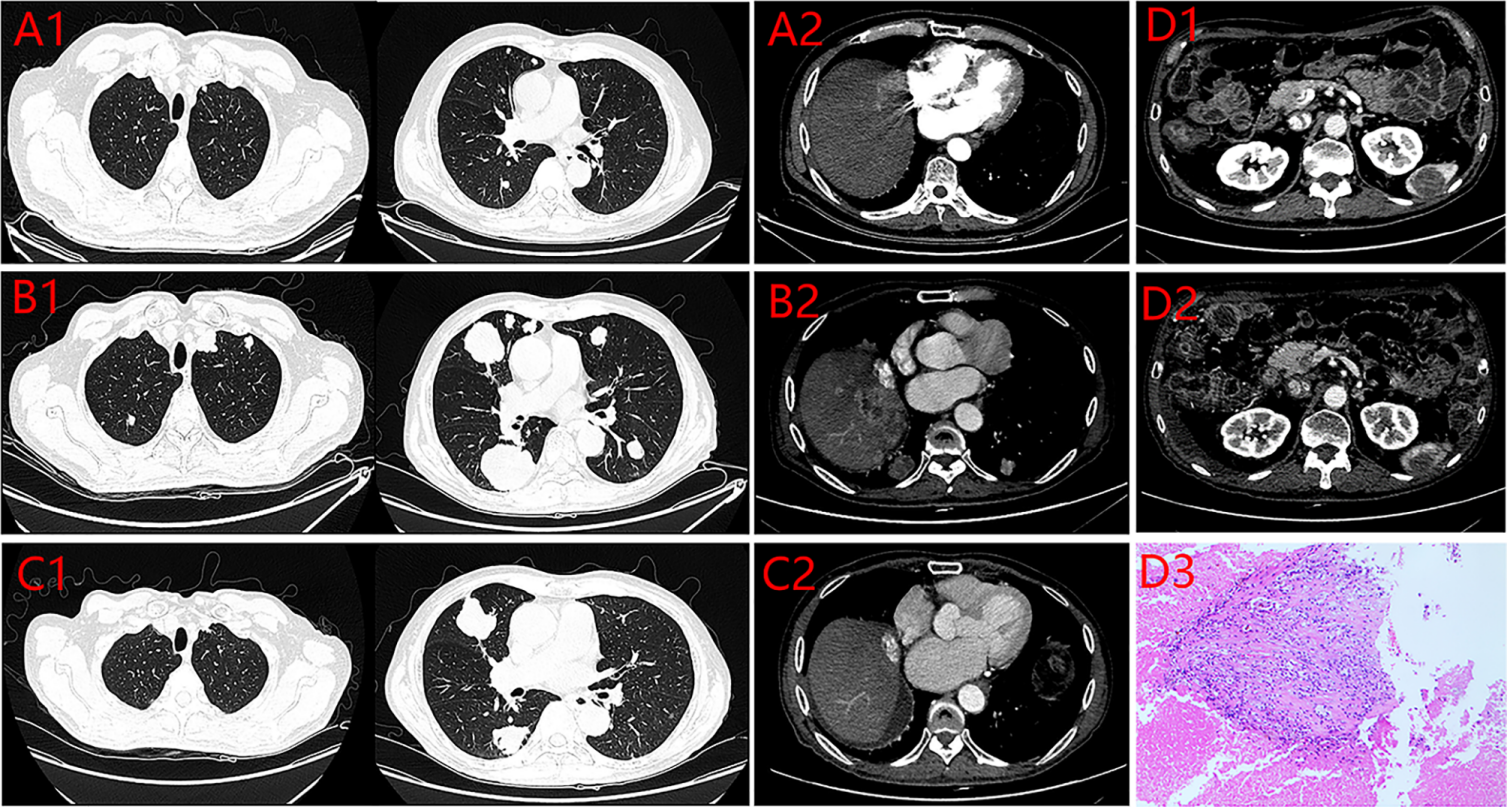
Enhanced CT of the lung and the abdomen. (A) 2017.09.17 (1. the Metastatic tumors of Lung 2. the Recurrent focus of Liver). (B) 2019.04.19 (1. The Metastatic tumors of Lung 2. the Recurrent focus of Liver). (C) 2019.11.11 (1. the Metastatic tumors of Lung 2. the Recurrent focus of Liver). (D) 1.spleen lesion (2019.4.19) 2. Spleen lesion after Pem plus Len (2019.11.11) 3. Postoperative pathology of spleen lesion.
